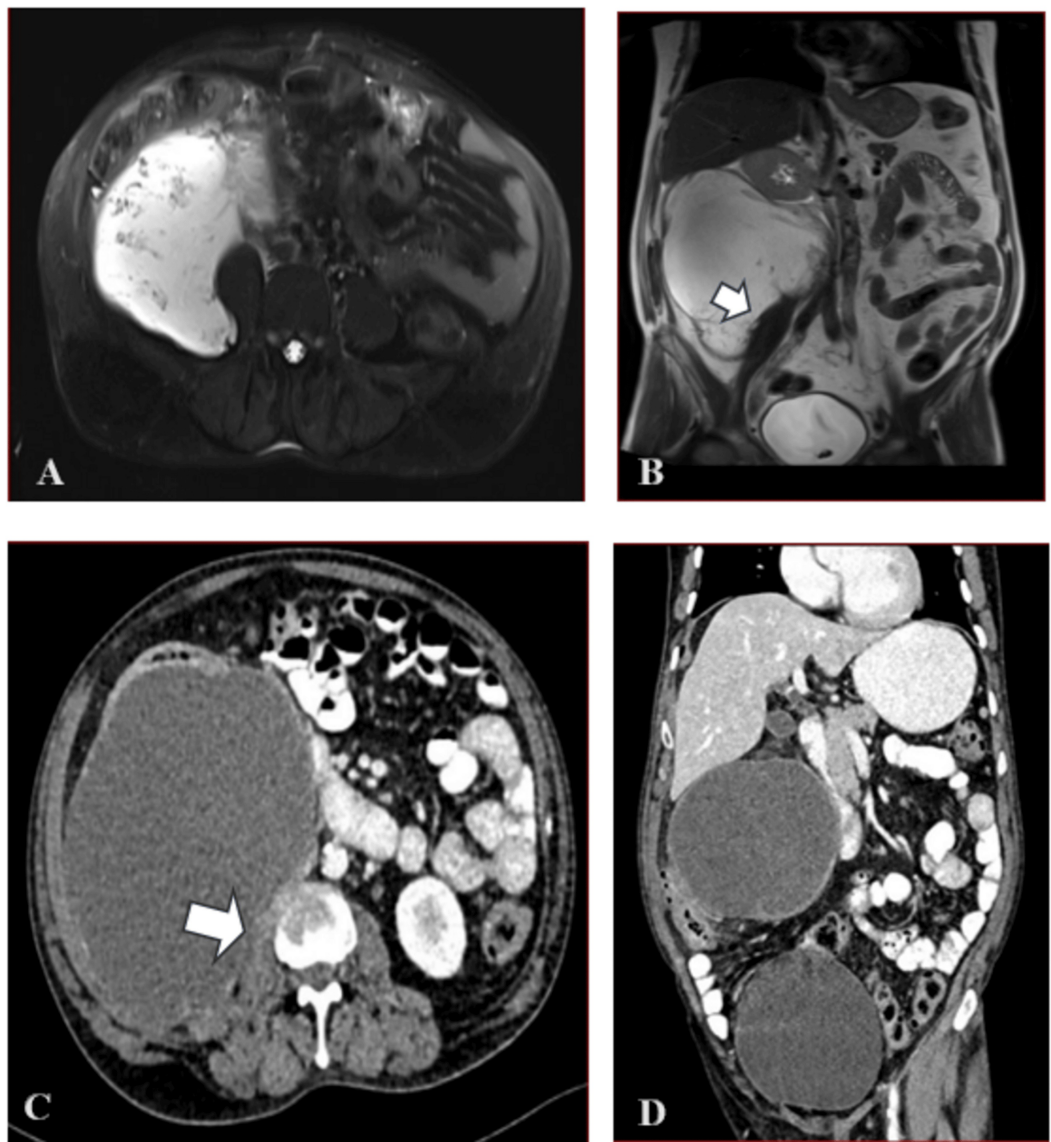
MRI and CT Images A) T2-weighted axial MRI image of the abdomen showing a hyperintense cystic lesion. B) T2-weighted coronal MRI image of the abdomen showing a large, well-circumscribed cystic lesion displacing the left kidney upwards (homogenous hyperintense). C) Contrast-enhanced CT of the abdomen showing a 16 cm × 15 cm homogenous cystic mass in the retroperitoneal space with displacement of the ascending colon with a solid component in the cyst wall  (white arrow). D) CT abdomen showing a well-circumscribed, homogenous hypodense lesion in the right hepatic and pelvic cavity.
